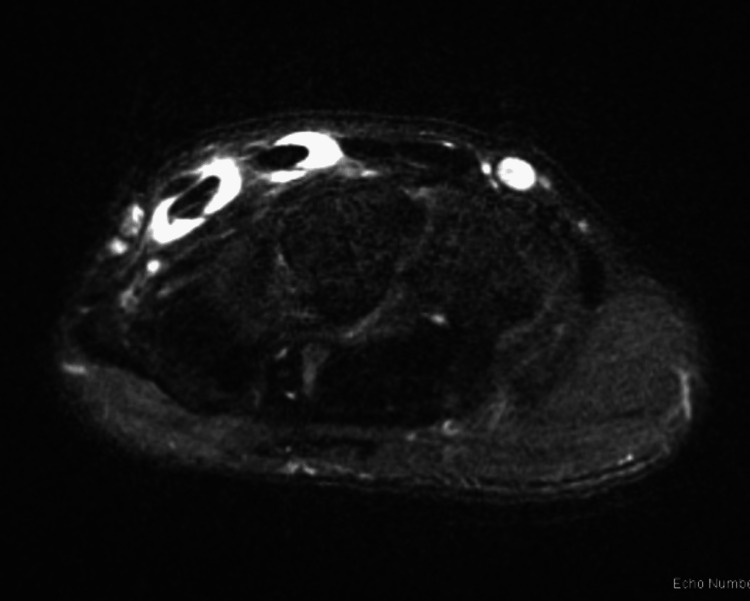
Axial T2-weighted images of the patient’s wrist demonstrating fluid within the tendon sheaths of the ECRB/ECRL and EPL ECRB: Extensor carpi radialis brevis; ECRL: Extensor carpi radialis longus; EPL: Extensor pollicis longus
